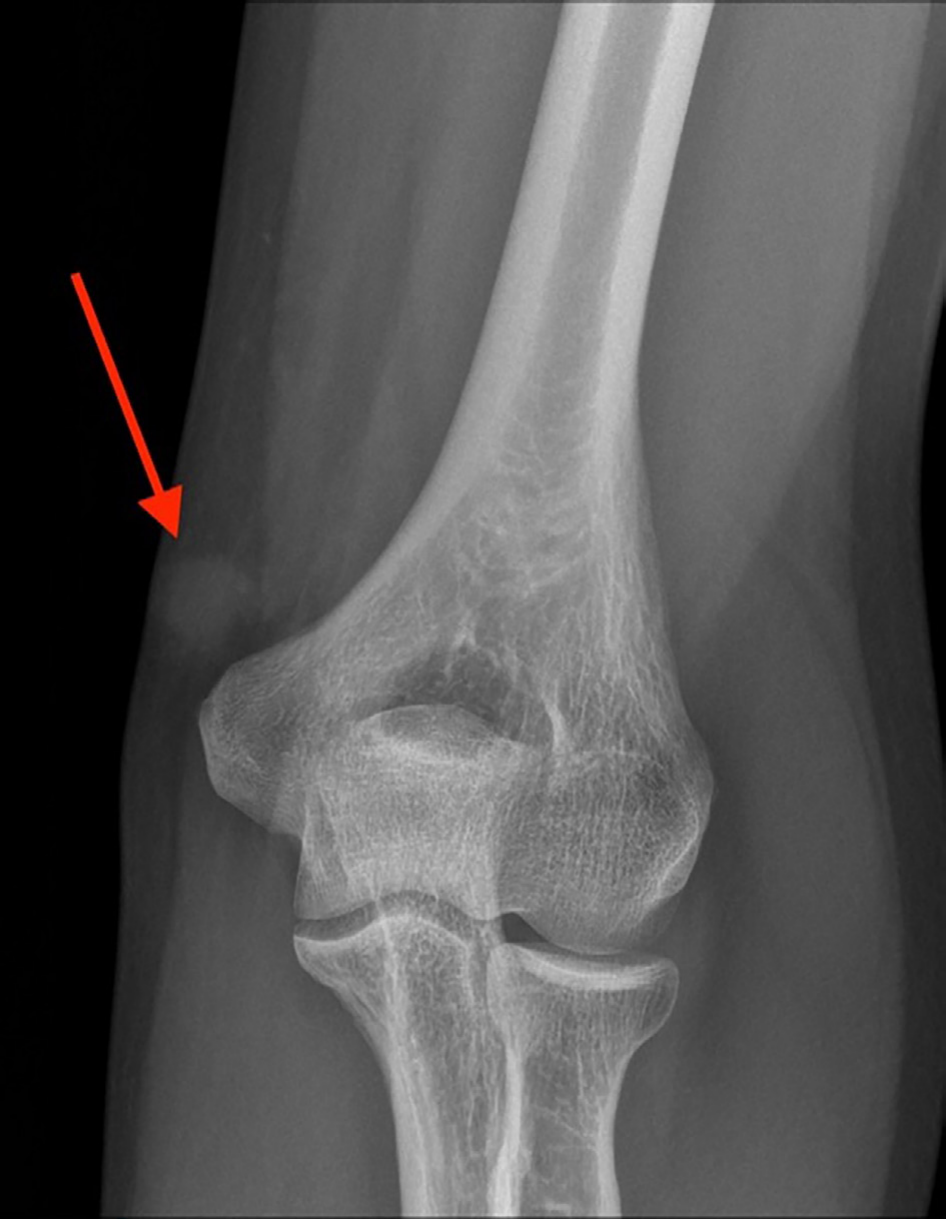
Conventional radiography of the left elbow reveals a nodular soft tissue opacity proximal to the medial epicondyle (arrow).
